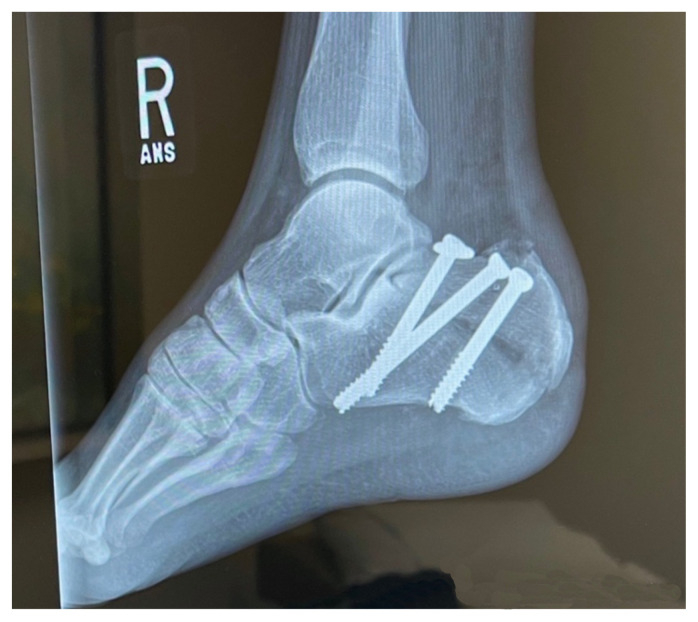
X-Ray After Initial Procedure
